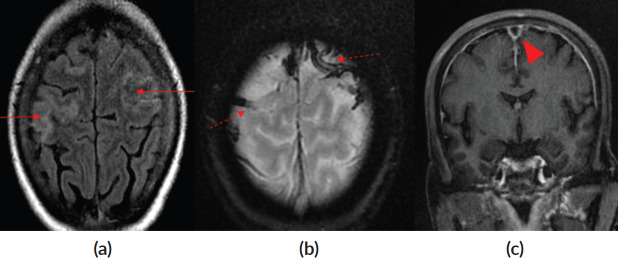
(a): Axial Fluid Attenuated Inversion Recovery (FLAIR) image depicting mild gyral oedema in bilateral high frontoparietal lobes (red arrows). (b): Gradient Recalled Echo (GRE) image showing blooming in cortical veins in bilateral high frontal lobes (red dashed arrows). (c): Post contrast T1 coronal image showing filling defect in superior sagittal sinus suggesting thrombosis (red arrow head).
